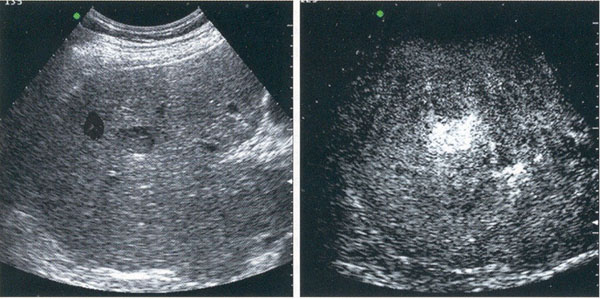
Left: Ultrasound (US) image of HCC. Right: contrast-US image of HCC with arterial enhancement (Courtesy Dr L. Biasi).
